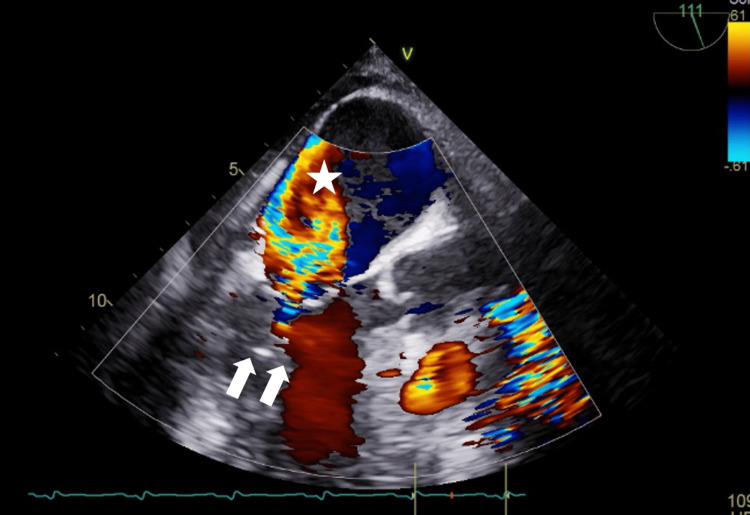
TEE findings after intraoperative heart displacement. Due to the narrowing of the left ventricular cavity caused by heart displacement, the tip of the Impella inlet (arrow) obstructed the supporting tissue of the posterior apex of the mitral valve, resulting in open fixation of the mitral valve and poor junction with the anterior apex, leading to severe MR (asterisk). TEE: transesophageal echocardiography; MR: mitral regurgitation
